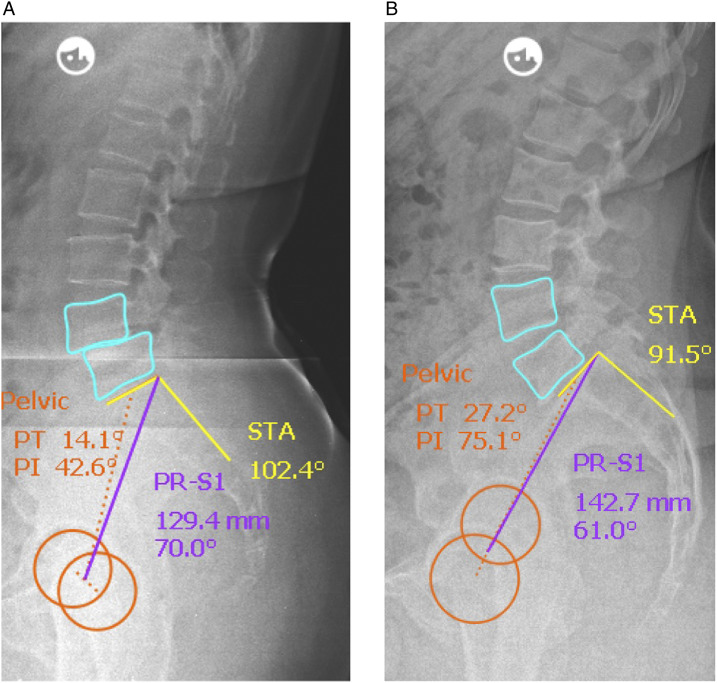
Radiographic examples of individual spinopelvic anatomy in sagittal radiographs for iSPL in L4/5 (a) and L5/S1 (b).
